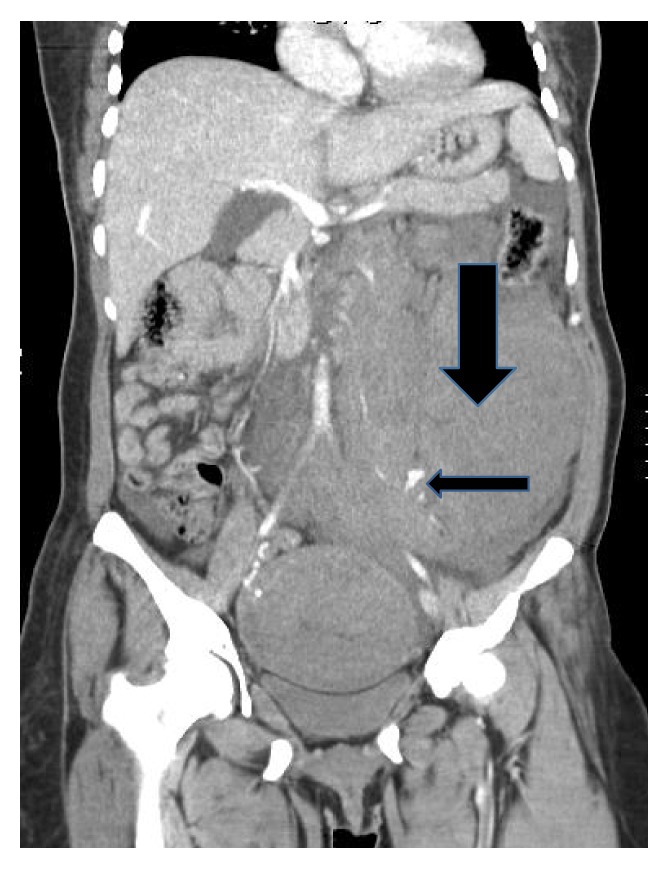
Retroperitoneal hematoma: coronal image of CT of the abdomen shows aneurysm in the left lower quadrant (narrow arrow), associated with a large left lower quadrant hematoma (bold arrow).
